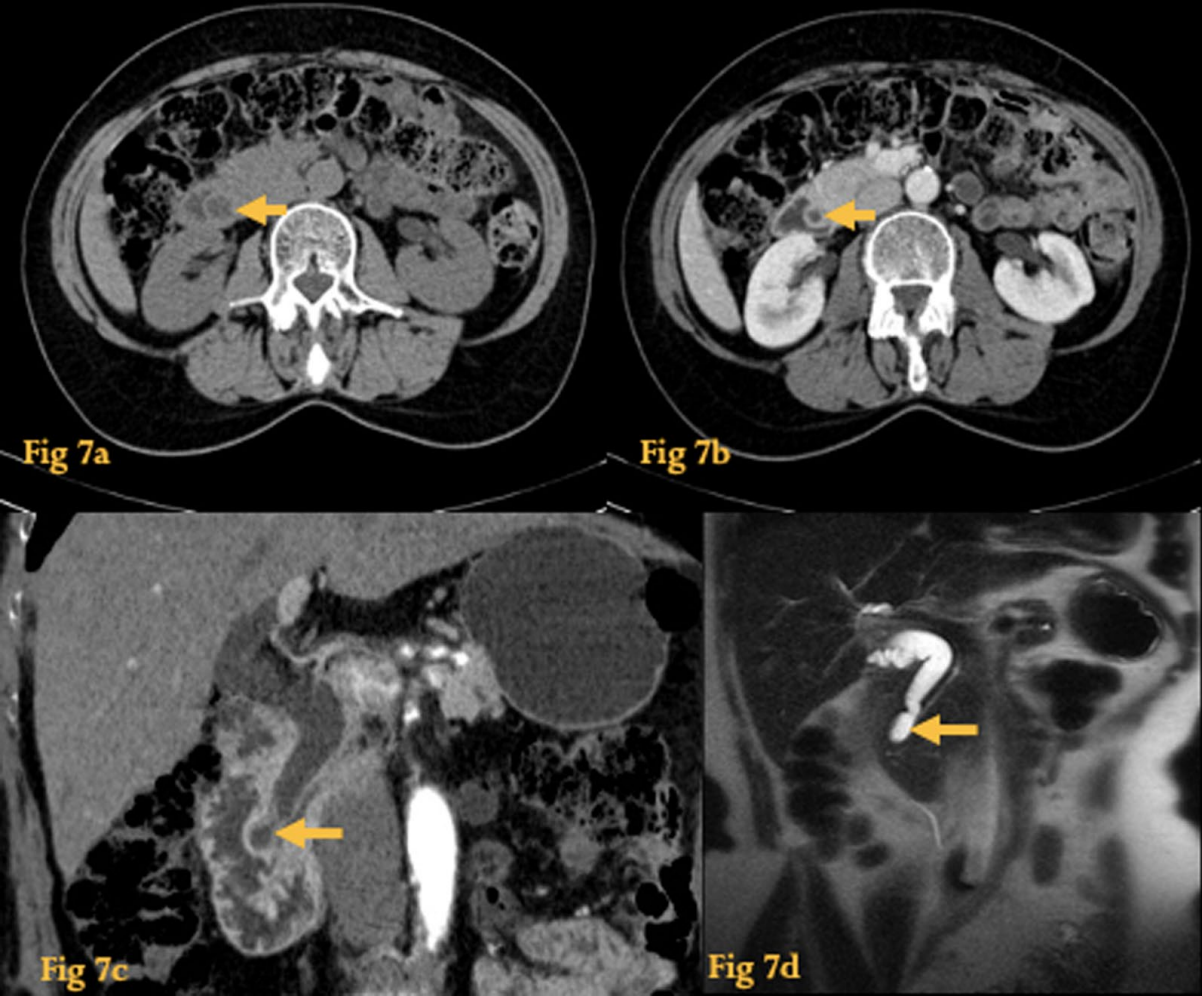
Choledococele. Unenhanced (a) and enhanced CT (b) shows a fluid-filled sac within the medial wall of the descending duodenum. CT coronal reformation (c) shows protrusion of a dilated distal segment of the common bile duct into the duodenum. Abdominal MR (d), coronal HASTE heavily T2-weighted sequence, shows distal CBD dilatation which protrudes into the duodenum
